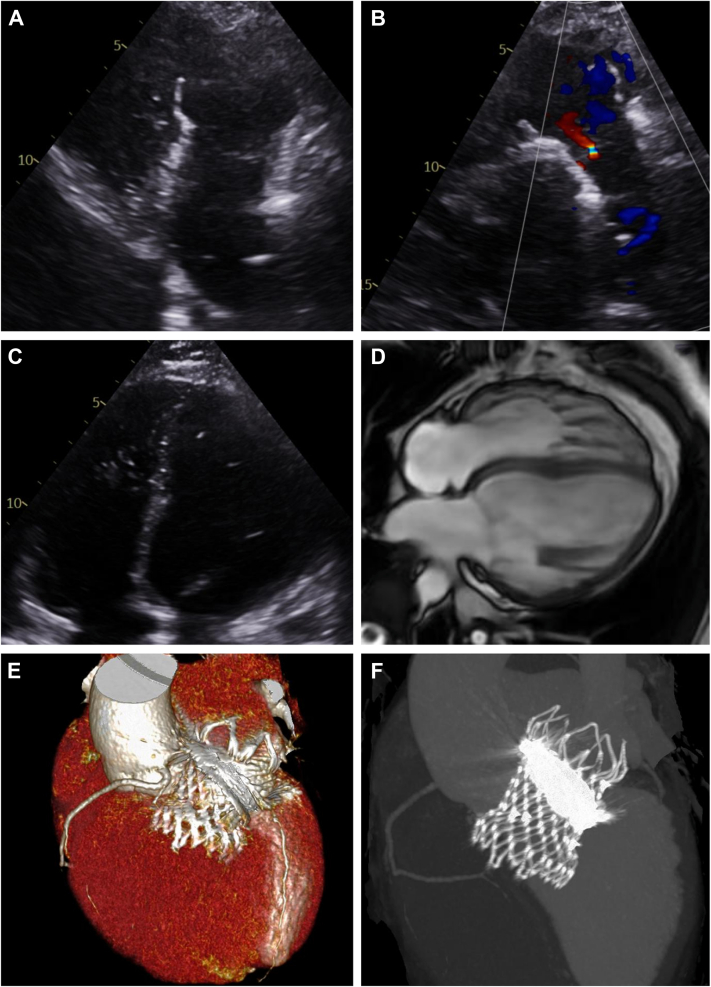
Follow-Up Imaging There were good results on the transthoracic echocardiogram at 3 months postprocedure with good position of the Venus-P valve in the modified RVOT view (a) and only minimal regurgitations demonstrated on colour flow and Pulsed wave Doppler (b) with favorable RV size reduction at 3 months by echocardiogram (c) and at 6 months by cardiac MRI (d). The CT obtained at 6 months shows the ring-like Cheatham Platinum stent and a Venus-P valve ensemble stable in the RVOT and main pulmonary artery in relation to the right and left coronary arteries (e-f). CT = computed tomography; MRI = magnetic resonance imaging; RV = right ventricle; RVOT = right ventricular outflow tract.
